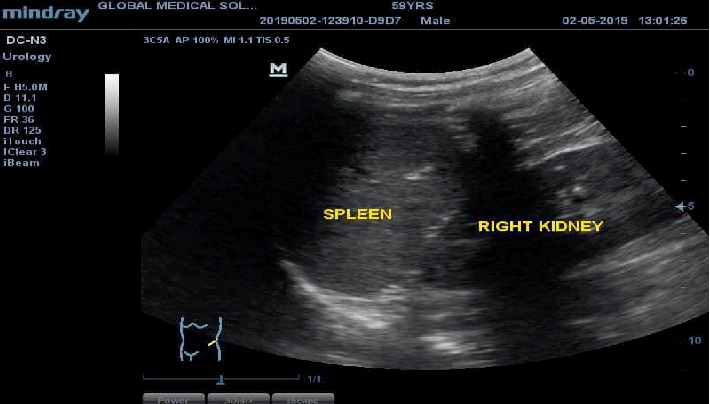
Sonogram of the right hypochondrium in longitudinal plane. The spleen is in the right upper quadrant under the right hemidiaphragm adjacent to the right kidney.
